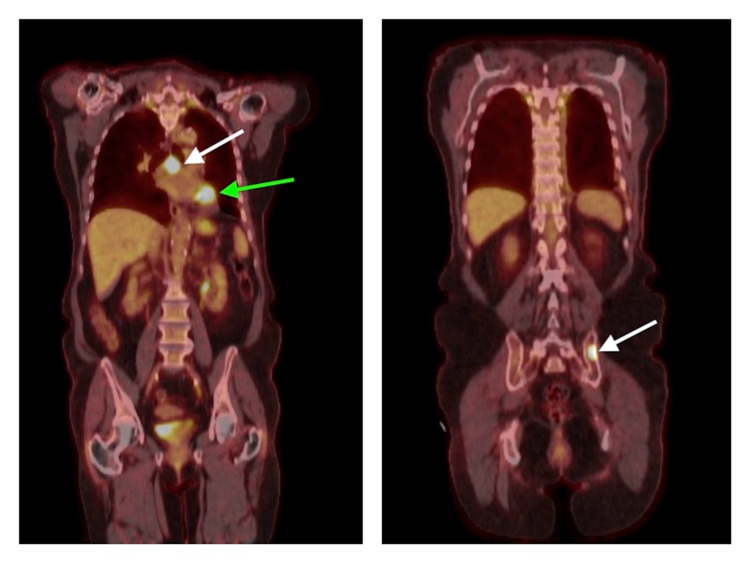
PET-CT scan from March 2020 Left: PET-CT scan from March 2020 following left lower lobectomy, demonstrating focal activity and soft tissue nodularity in the left hilar surgical site (indicated by green arrow) as well as metabolically active subcarinal lymph nodes (indicated by white arrow), concerning for metastatic disease. Right: PET-CT scan from March 2020, which revealed focal activity in the left ilium (as indicated by the white arrow), concerning for osseous metastatic disease. PET-CT: positron emission tomography-computed tomography.
